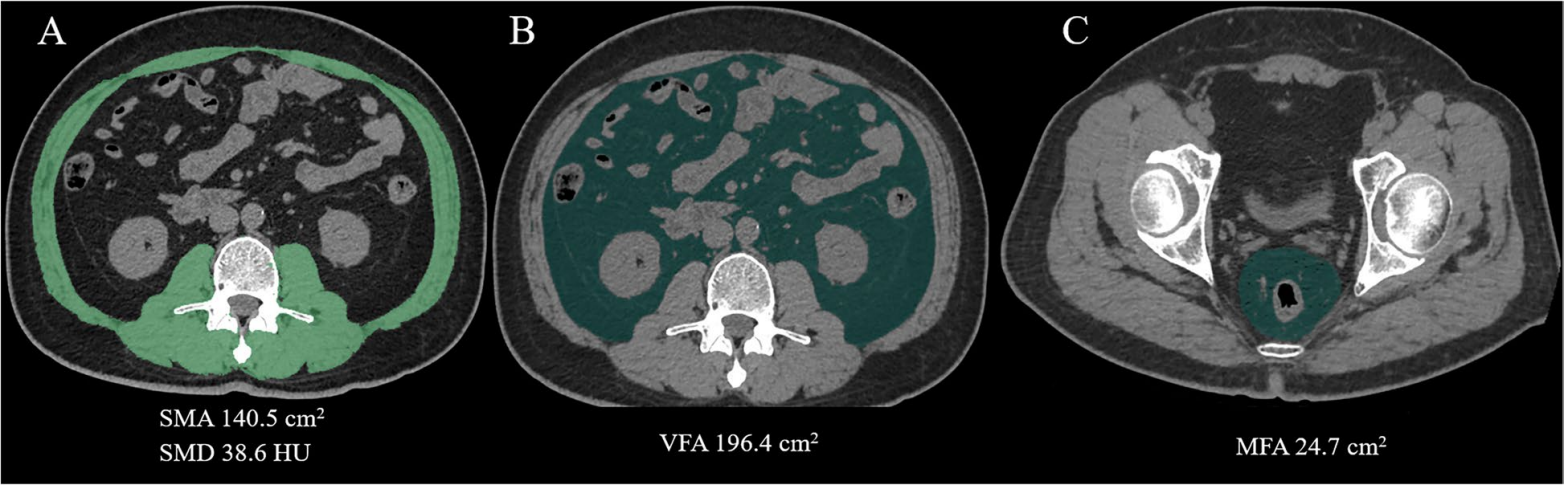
Abdominal axal CT images of patients with quantitative measurements of SMA, SMD, VFA at the level of L3 and MFA at the level of ischial spine
